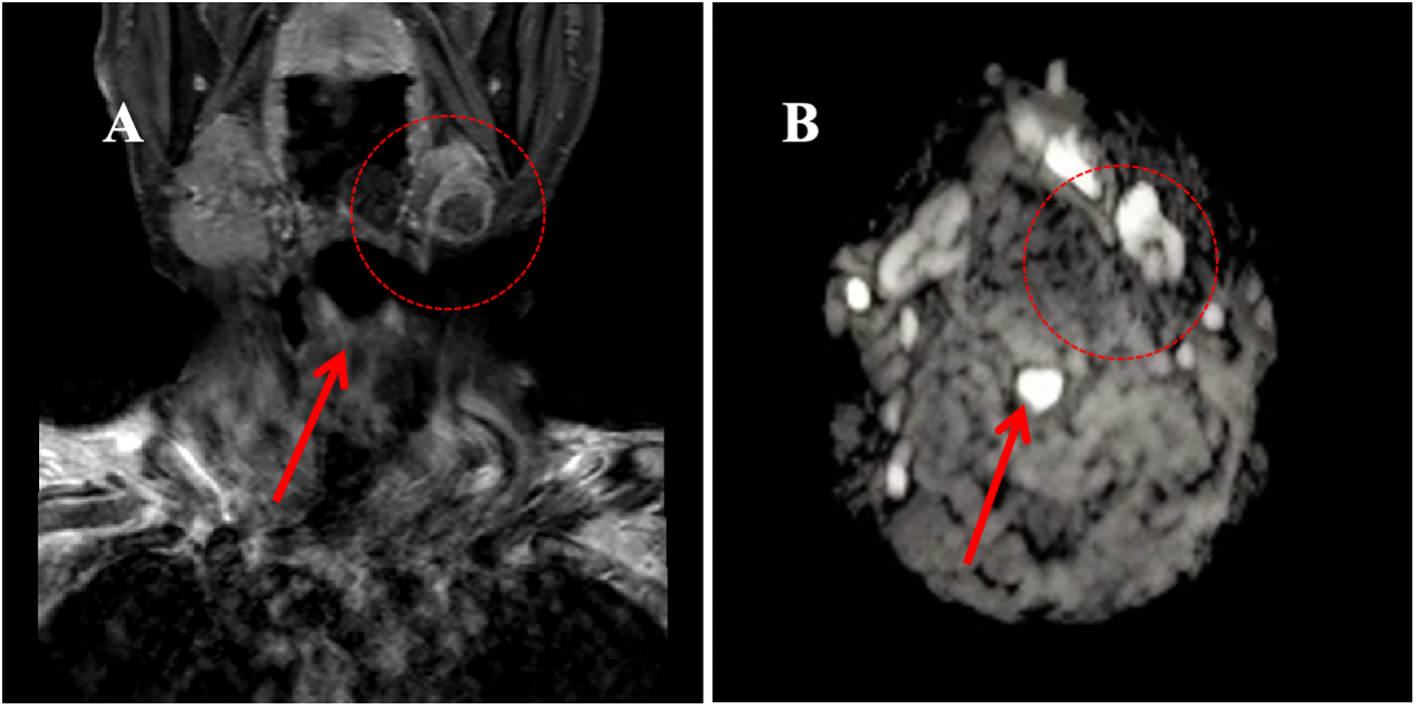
Magnetic Resonance Imaging (MRI) of the neck region in June 2020. (A) T1-weighted image showing a hypointense lesion in the left submandibular gland (approximately 1.5×1.3×1.1 cm); (B) Diffusion-weighted image demonstrating a hyperintense signal in the left submandibular gland.
